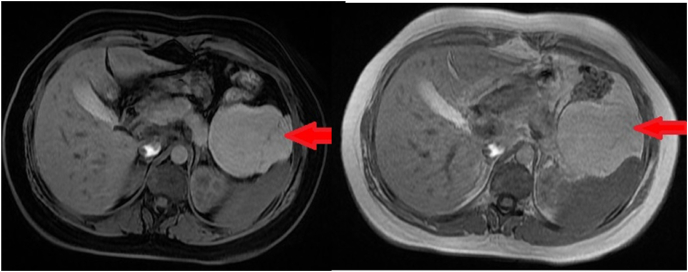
Abdominal MRI showing an intraperitoneal cystic mass located at the level of the left hypochondrium presenting hypointensity in the T1 weighted and hyperintensity at T2 weighted with some fine partitions.
